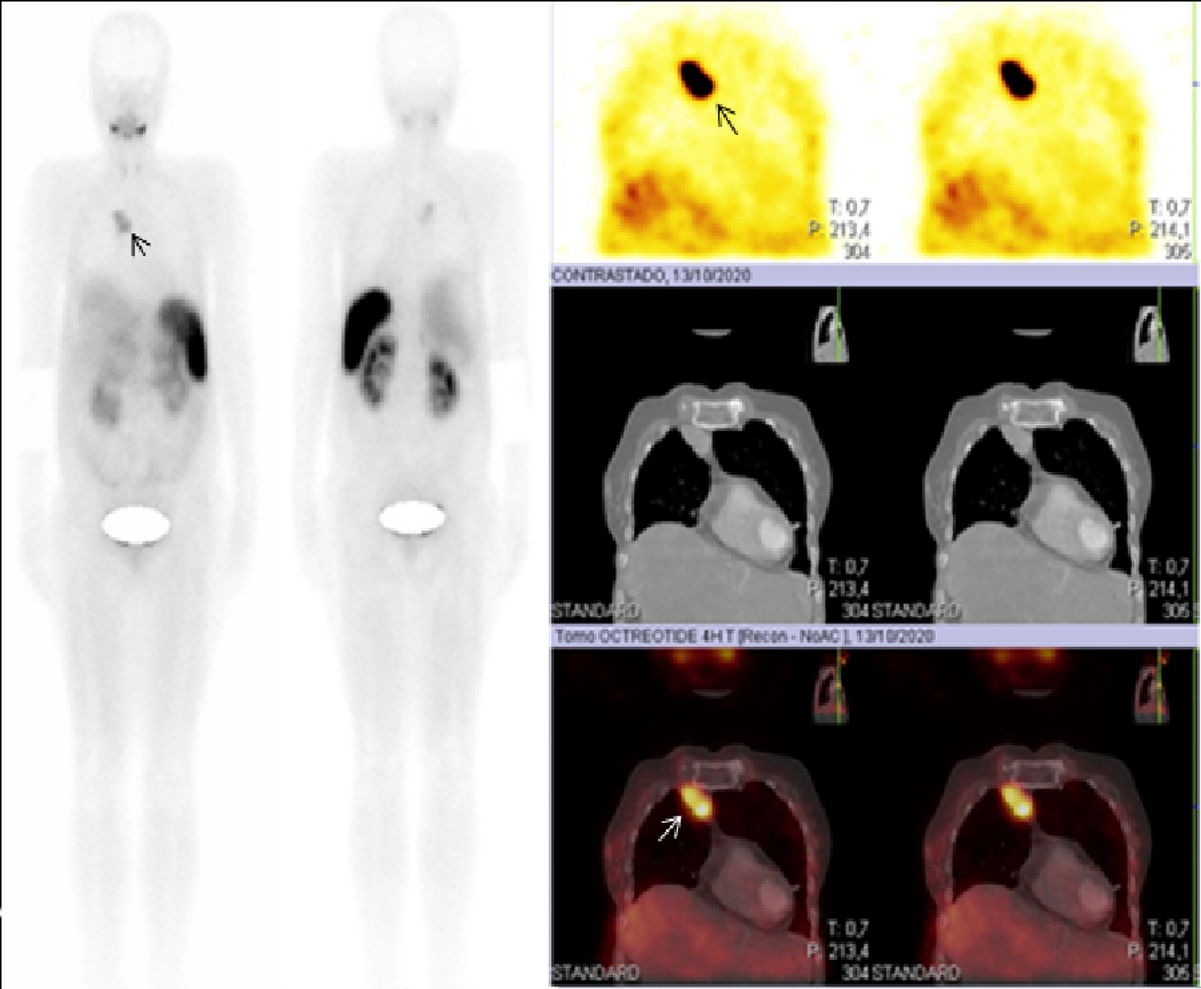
OctreoScan (also known as Somatostatin receptor scintigraphy). Positive for tumoral uptake with somatostatin receptors in thorax, with no evidence of liver uptake.
